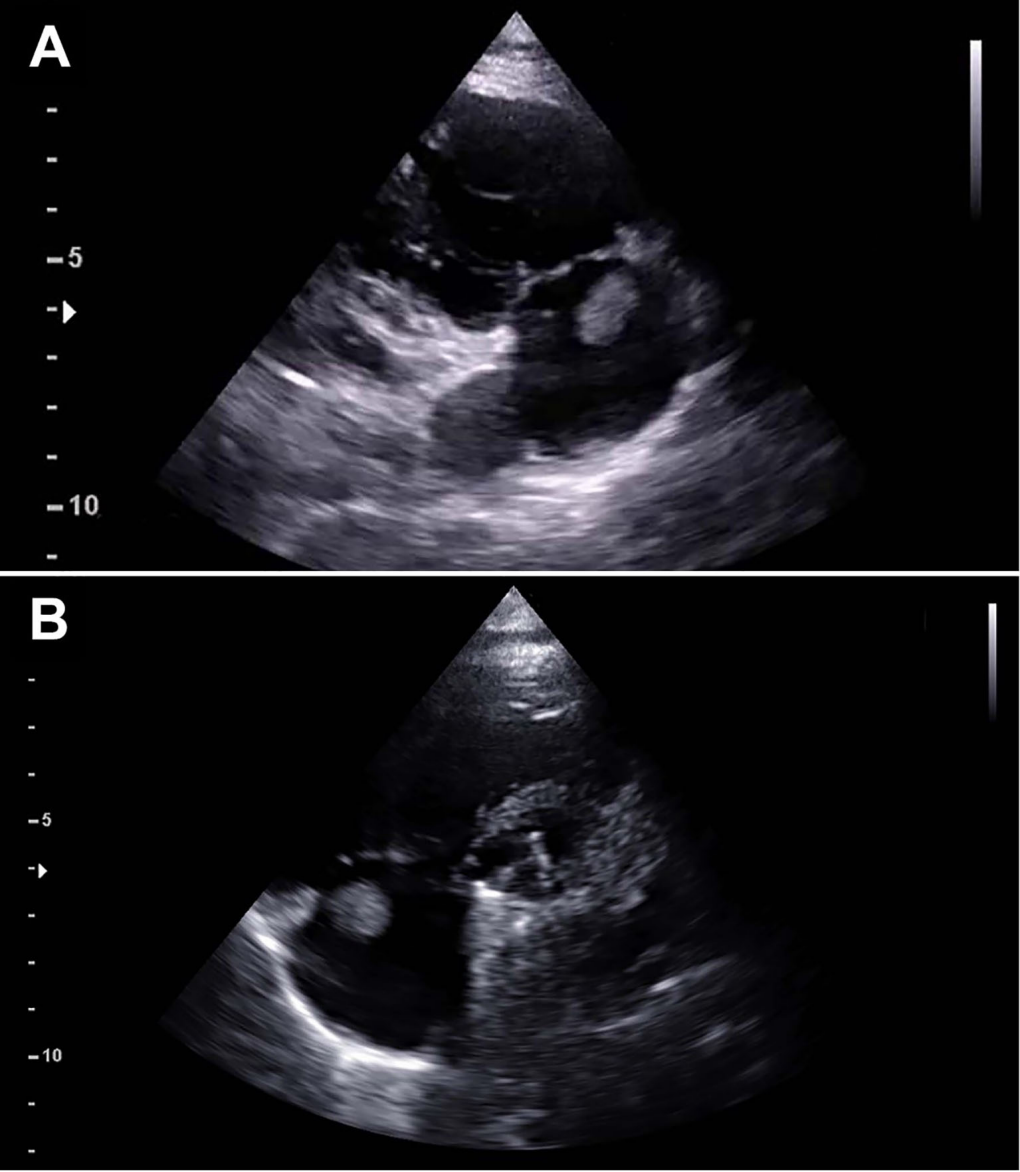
Preoperative echocardiography. Preoperative echocardiography demonstrating a mass measuring 11×12 mm attached at the junction of the right atrium and tricuspid annulus as shown in the right ventricle inflow view (A) and parasternal short axis view (B).
